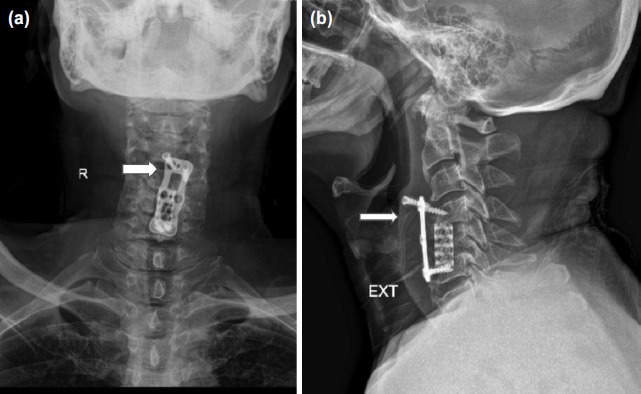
Radiographs of cervical spine with (a) AP view showing back-out of right sided proximal screw of cervical plate as denoted by white arrow with plate shifted to left, (b) lateral extension view showing one of proximal screws completely backed-out, marked with white arrow of anterior cervical plate with other one partially backed-out.
